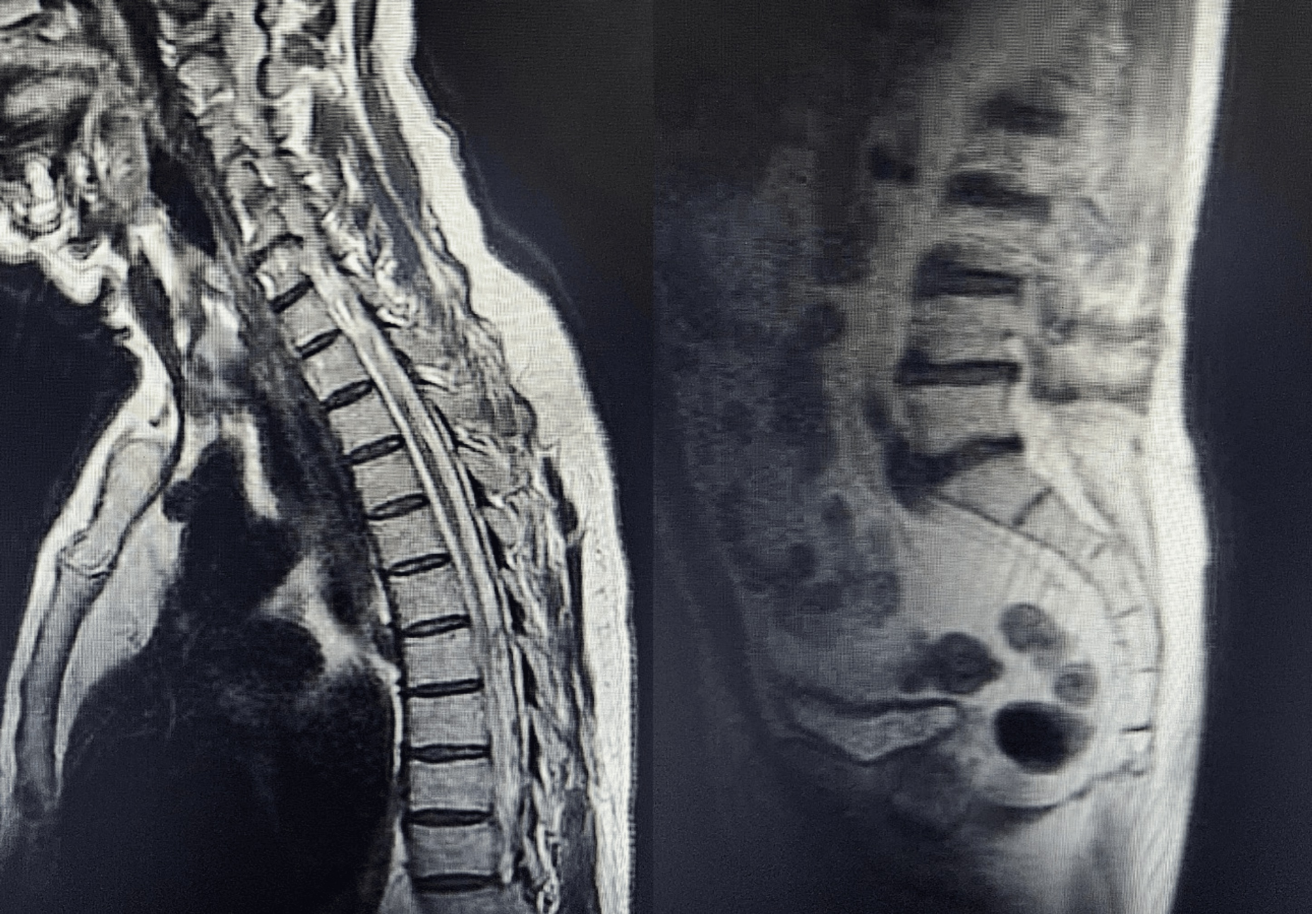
MRI of the thoracic (left) and lumbar (right) spine Thoracic T2-weighted imaging demonstrated a subtle non-enhancing T2-hyperintense mass-like area along the dorsal aspect of the cord extending from T5-6 to the superior endplate of T8 with effacement along the posterior aspect of the cord. Given the lack of enhancement, a mass was considered unlikely. Lumbar spine imaging showed no acute fracture, subluxation, or marrow-replacing process. A linear hyperintensity was seen along the ventral aspect of the distal cord and cauda equina, possibly artifactual. MRI: magnetic resonance imaging
